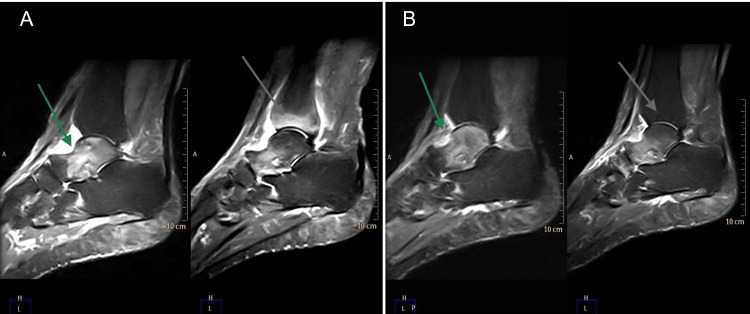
Magnetic resonance imaging of tibiotarsal joints before (A) and after (B) the treatment with neridronate, with improvement of bone edema (grey arrow) and synovitis (green arrow).
